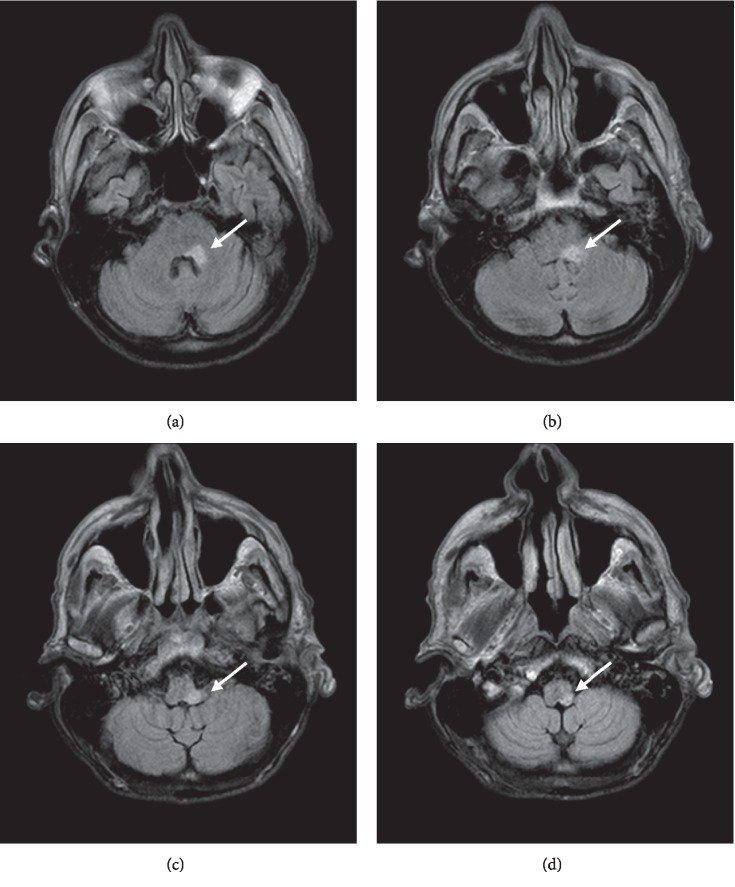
FLAIR (fluid-attenuated inversion recovery) images. (a) Focus of increased signal intensity noted at the level of the fourth ventricle involving the left CN VI (abducens) nucleus, left CN VII (acial) nucleus, and left middle cerebellar peduncle. (b) Focus of increased signal extends caudally to the level of the cerebellar vermis involving the middle cerebellar peduncle. (c) Further to the level of the cerebellar vermis with involvement of the medulla oblongata and left pyramid. (d) Terminating at the level of the cerebellar tonsils involving the left inferior cerebellar peduncle (restiform body).
